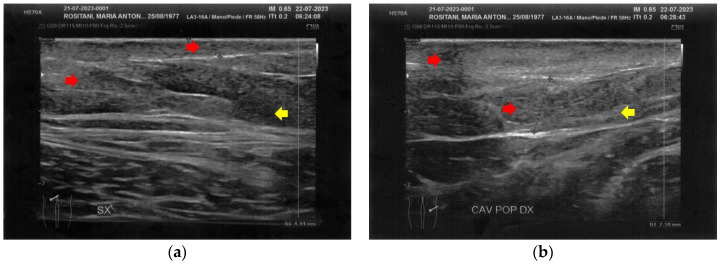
Ultrasonography of the popliteal fossae at T1. (a) The left side presents a clear improvement in both the cicatricial fibrosis (red arrows) and the reorganization of the different structural layers (yellow arrow); (b) The right side presents the same effects of the treatment noted for the left side.
